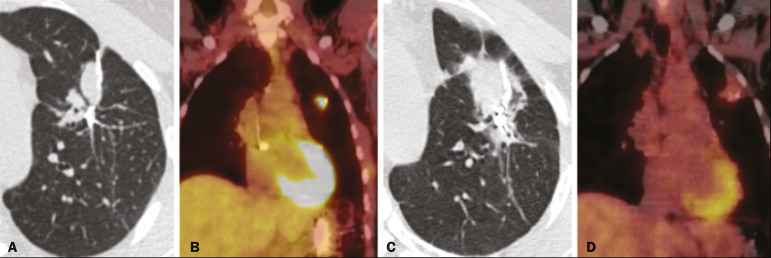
Lung metastasis from an adrenal carcinoma, shown in a CT scan (A), as well as in an 18F-FDG PET/CT scan (B), on which the standardized uptake value (SUV) was 6.1. C,D: Follow-up images acquired at 15 months after treatment with stereotactic radiotherapy, showing that the patient had developed mass-type radiation-induced fibrosis (CT scan in C), with an SUV < 5 (18F-FDG PET/CT scan in D).
